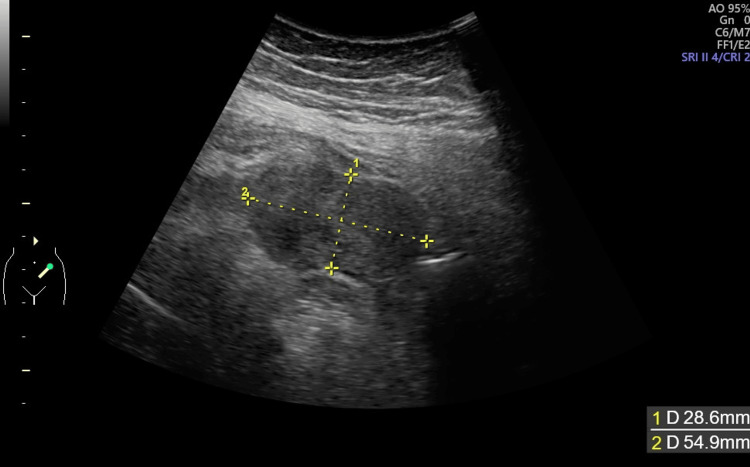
Transabdominal ultrasonography. A transabdominal ultrasound showing a 54 × 28 mm mass in the left lower abdomen.
